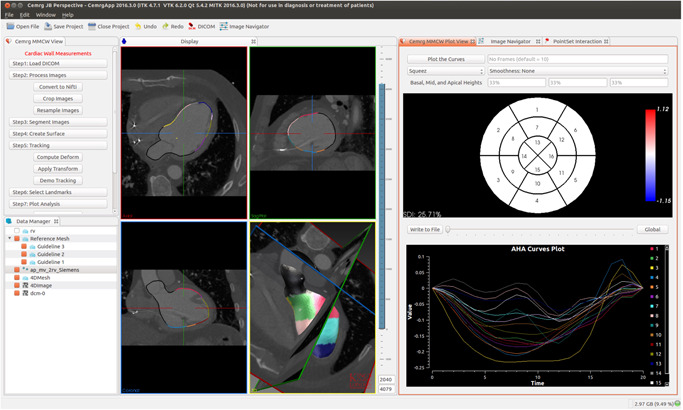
Cardiac computed tomography (CT) yssynchrony analys is platform based on open‐source software medical imaging interaction toolkit (MITK) provides a simple stepwise approach for tracking wall motion from cardiac CT datasets. (A) Interactive image rendering for visualizing 3D images and surface meshes. (B) 16‐segment bullseye plot for visualization of myocardial strain. (C) Individual strain curves; each color represents an American Heart Association (AHA) segment
